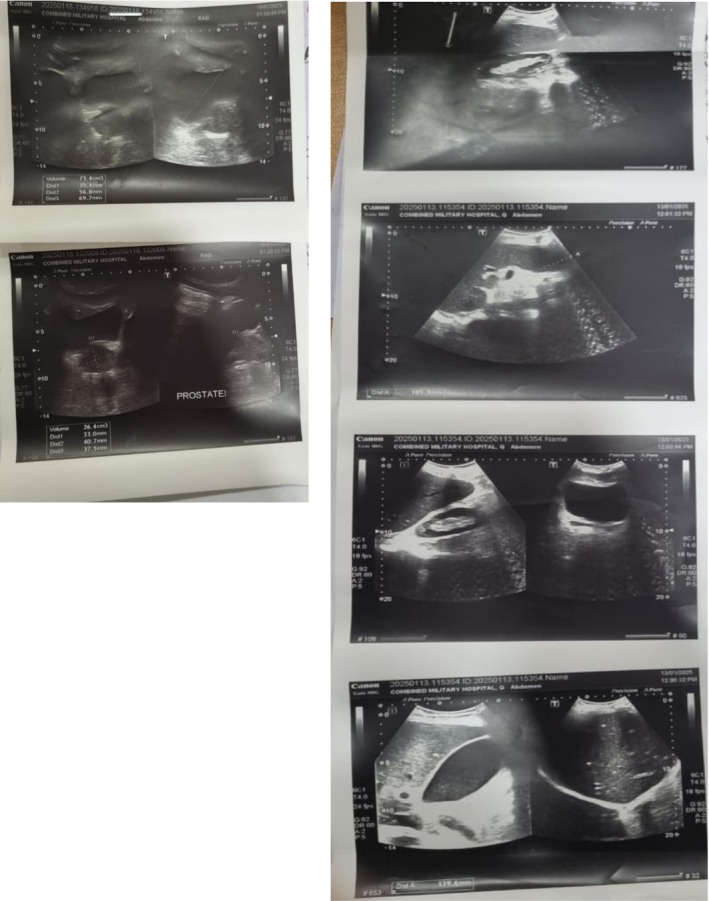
Ultrasound (USG) of the abdomen and prostate (pre and post void urine). Transverse view showing splenomegaly (spleen measuring 18.5 cm, arrow indicating spleen border) with normal‐sized kidneys exhibiting increased parenchymal echogenicity. Mildly enlarged prostate (26 cm^3^) with post‐void residual volume of 70 mL is noted. No ascites or focal lesions observed. (Image courtesy of Combined Military Hospital Quetta; patient identifiers removed).
